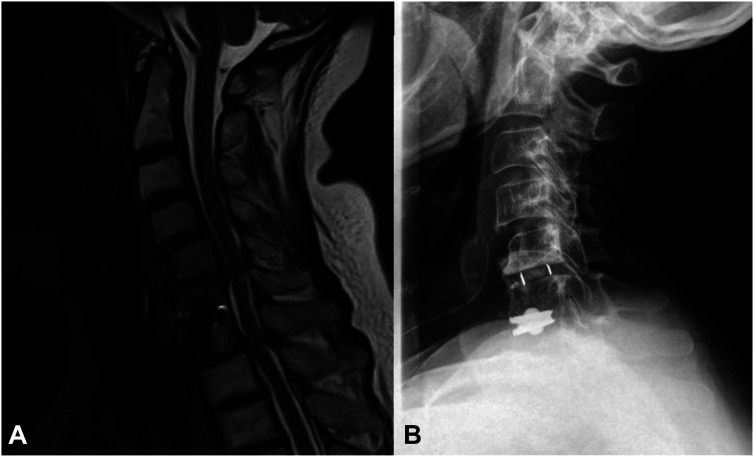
A 52-year-old female patient, who was treated seven years ago with a disc prosthesis based on the technical design “ball and socket” in C6/C7. The reason for a prosthetic restauration is to protect the adjacent segments. (A) MRI scan of the cervical spine: the adjacent segments C5/C6 and C7/Th1 are subject to considerable degenerative changes. (B) Due to myelopathy and cervical stenosis, C5/6 is implanted with a cage. C7/Th1 also requires surgical treatment.
